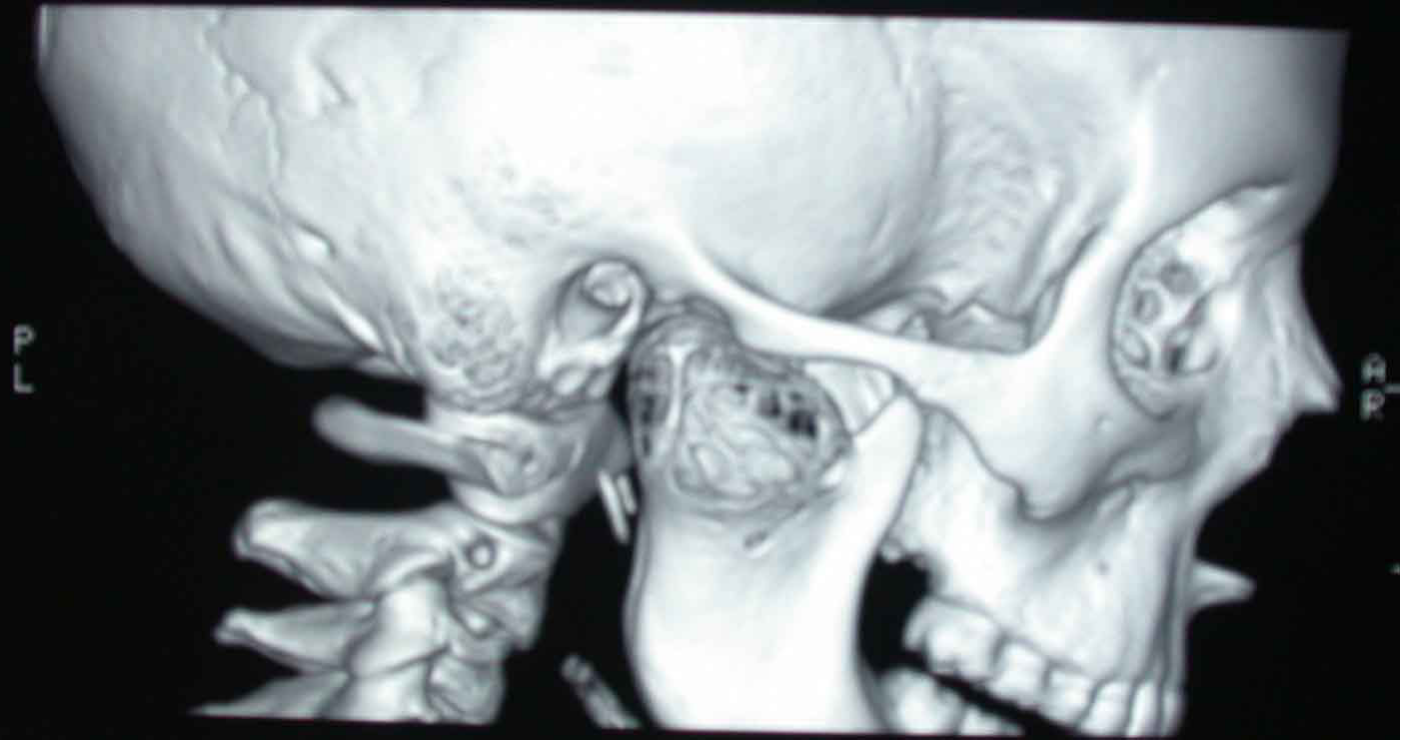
Three-dimensional CT reconstruction showing the presence of a radiolucent and multilocular lesion in the condyle region, the coronoid and part of the ramus. Note the honeycomb appearance and septae within the bony lesion.
